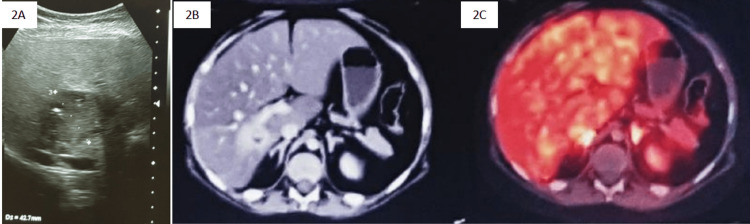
Ultrasonogram showing mixed echogenic lesion measuring 54 × 43 × 41 mm with internal cystic areas and calcification (2A); 68Ga-DOTANOC PET/CT showing an ill-defined enhancing mass measuring 41 × 30 mm in segment VII and VI of the liver with a small central area of hypodensity, preserved perilesional fat, and persistent enhancement on delayed phase (2B) that was DOTANOC non-avid (2C) suggestive of hemangioma.
